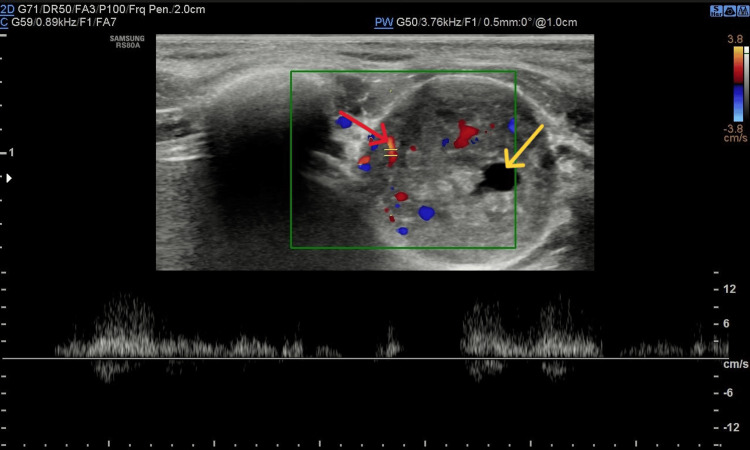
Image from Doppler ultrasonography: lesion with arterial flow (red arrow) and cystic elements (yellow arrow) is presented
